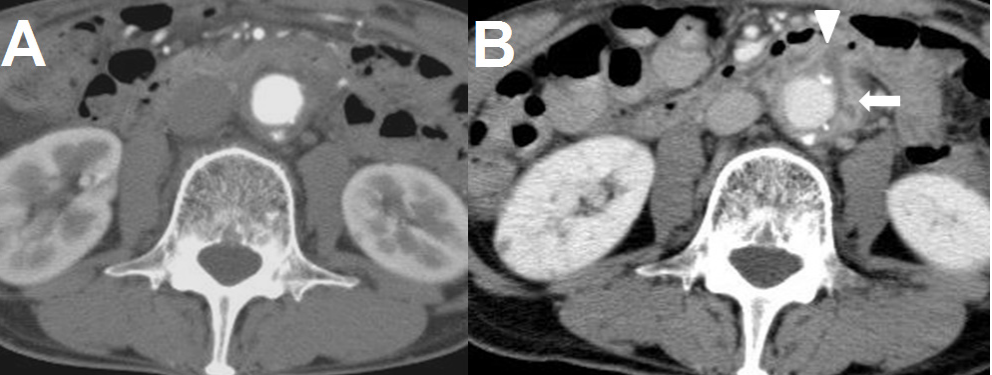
A CT scan at 2 months after the aortic surgery. No abnormalities were seen around the aorta. B CT scan at the fourth day after patient admission. Low-density area(*arrowhead*) had increased between the graft wall and the aneurismal wall(*arrow*), and seemed to continue to the duodenum.
